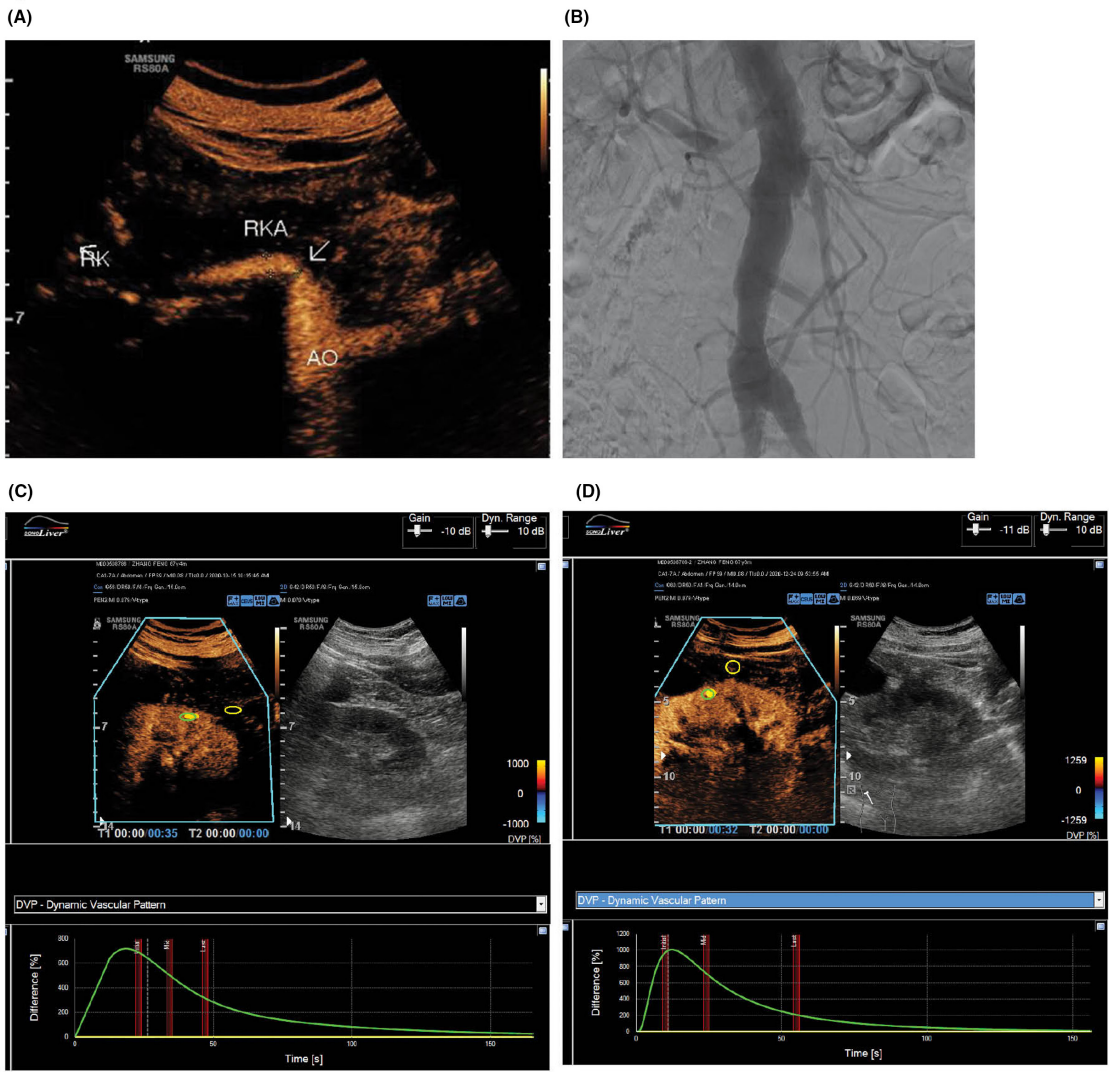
Routine ultrasound and CEUS (A) and DSA (B) showed 85% localized stenosis of the right renal artery with impaired renal cortical blood perfusion (C), which significantly improved after stent implantation (D).^6^
